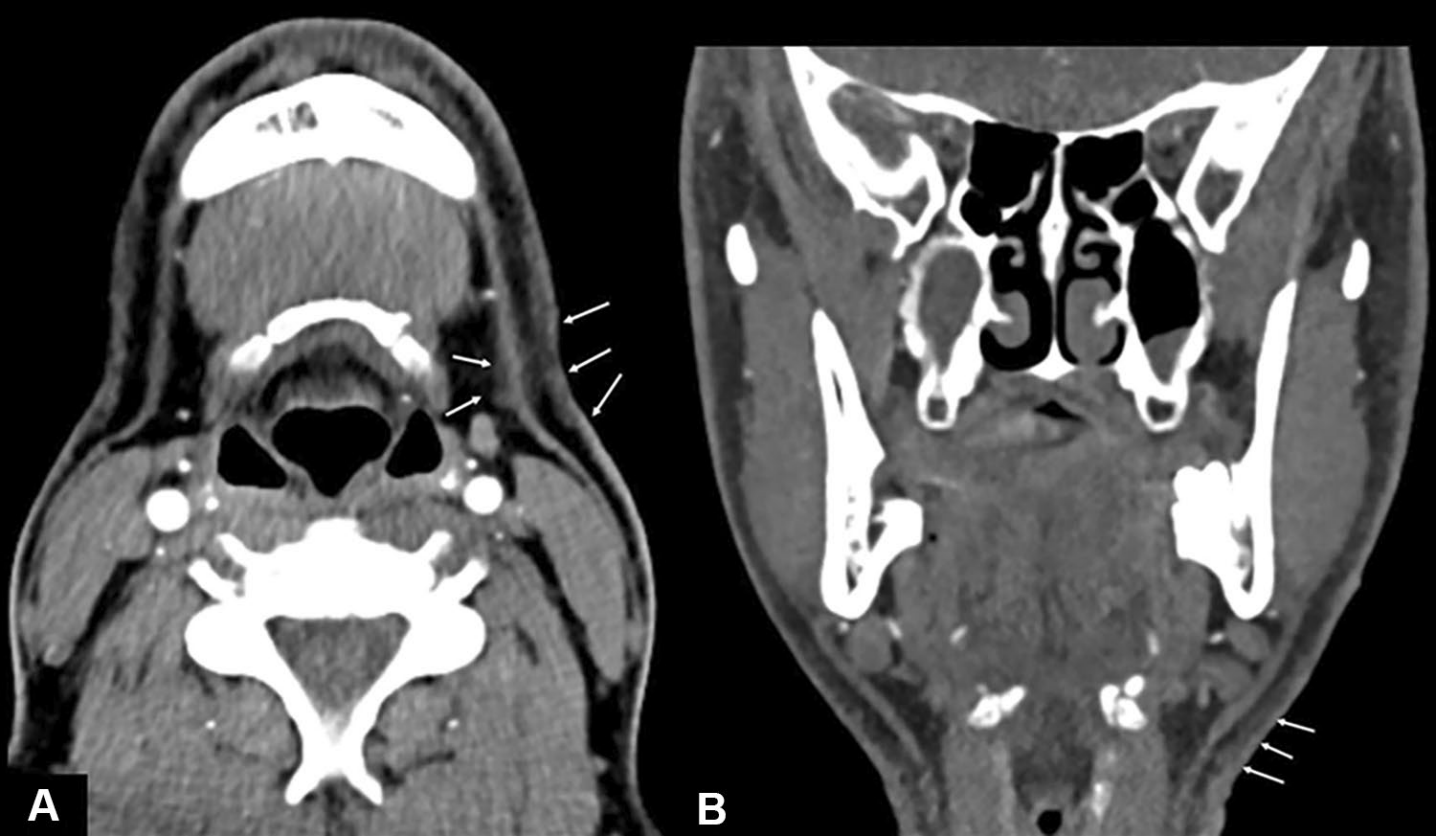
Axial and coronal non-enhanced CT (NECT); soft-tissue window with a slice thickness (ST) of 3 mm. The axial (A) and coronal (B) NECT images show a subtle stranding of the subcutaneous fat in the left submandibular region and a slight thickening of the cutis (arrows)
